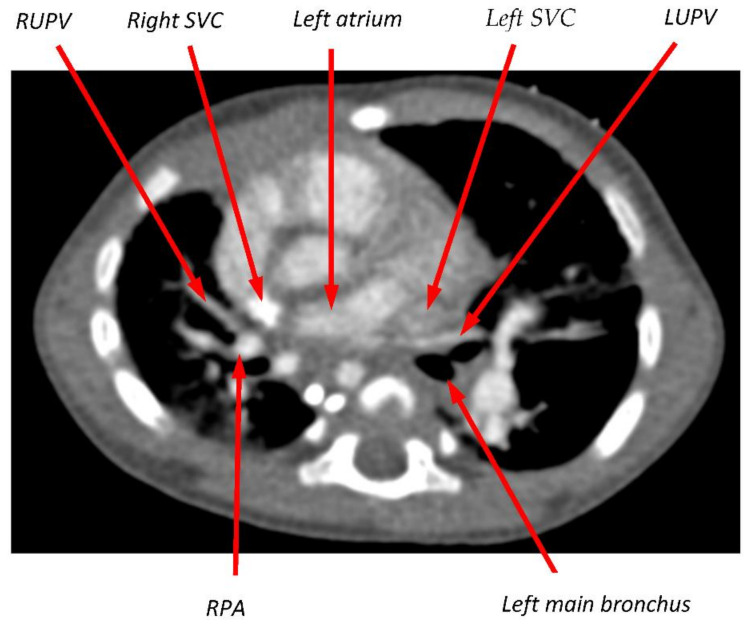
Transverse slice of CT scan from Pt2 at the level of the left atrium (LA) and upper pulmonary veins. The RUPV was documented as atretic at the time of this scan, but its course had presumably been between the right superior vena cava (SVC) and the right pulmonary artery (RPA). On the left side, the takeoff of the LUPV from the LA is sharply angulated, and the vein appears compressed as it passes between the left SVC and the left main bronchus.
